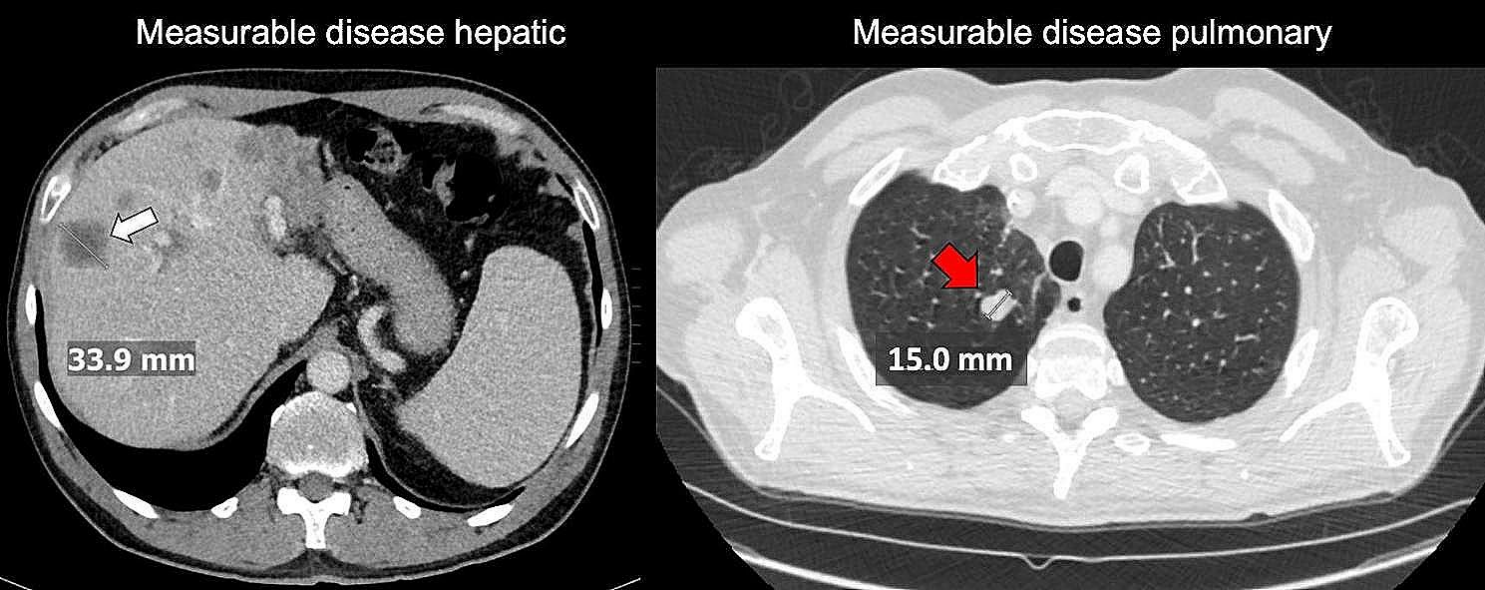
Exemplary cases of MD representative of the overall tumor burden in contrast enhanced CT Left image: Patient with recurrent colorectal cancer after surgery with multiple metachronous liver metastases. An axial CT-image of the liver in soft tissue window displays a well-defined liver metastasis in liver segment VIII is measured (white arrow). Right image: Axial CT image in pulmonary window in a patient with resected sarcoma and well-defined measurable pulmonary metastases. A well-defined measurable lesion in the right upper pulmonary lobe is displayed (red arrow, 15 mm)
